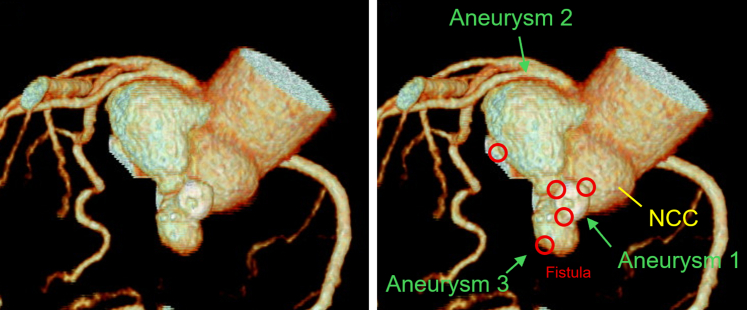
The Spatial Relationship Between the Aneurysm and the Fistula on 3D CT Reconstruction Systolic flow from the aorta entered the NCC-side aneurysm through a 3-mm-diameter fistulous connection. This aneurysm subsequently communicated via 3-mm-diameter fistulas with both the left ventricular outflow tract (LVOT)-side 10-mm-diameter aneurysm and the LCC-side 14-mm-diameter aneurysm, each of which also maintained communication with the LVOT.
